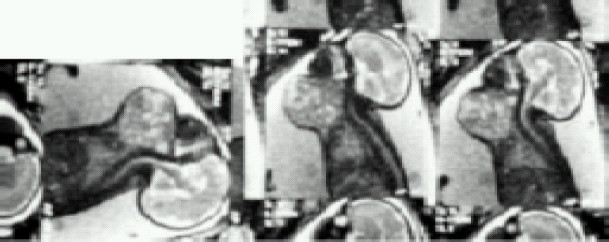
Intrauterine appearance of fetus in CT
